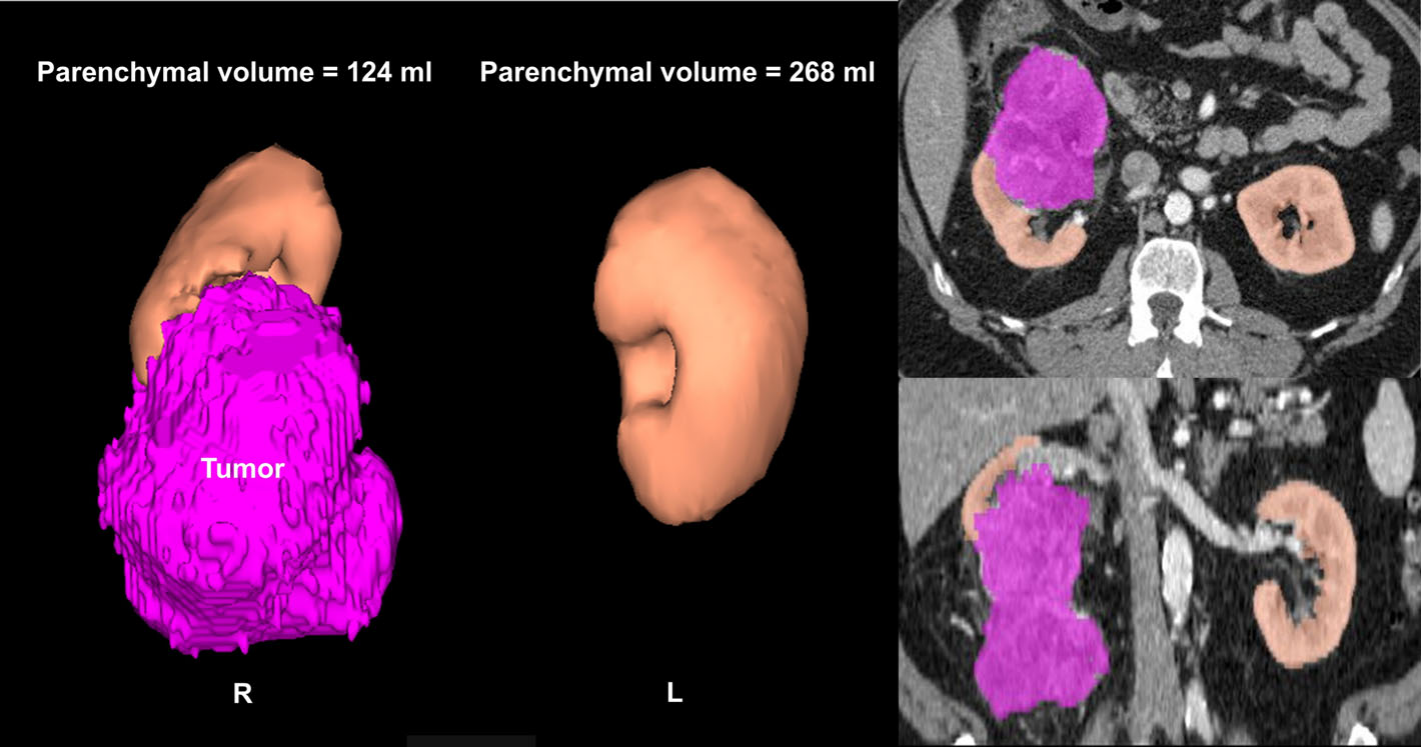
Estimation of parenchymal volume replacement (PVR). Parenchymal volume replacement was estimated using semi‐automated software with the tumour shown in purple and the parenchyma in beige. The images on the left show three‐dimensional views of the kidneys and on the right images from the transverse and coronal sequences with parenchymal volumes overlayed on the standard CT image. Percent PVR is defined as parenchymal volume_contralateral_ − parenchymal volume_ipsilateral_ normalized by parenchymal volume_contralateral._
